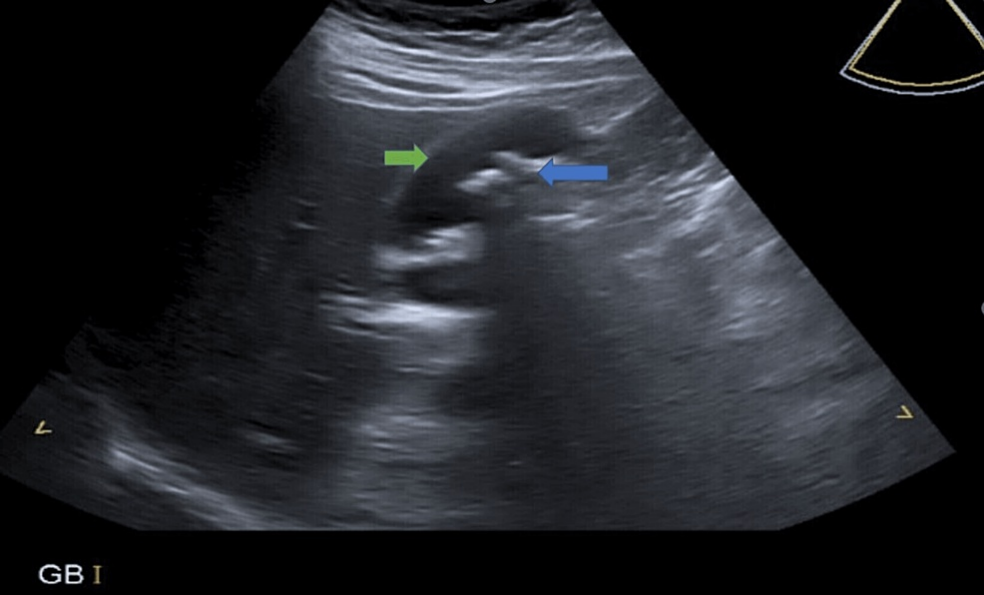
Ultrasound showed a thickened gall bladder wall (green arrow) with multiple stones in gall bladder (blue arrow)
